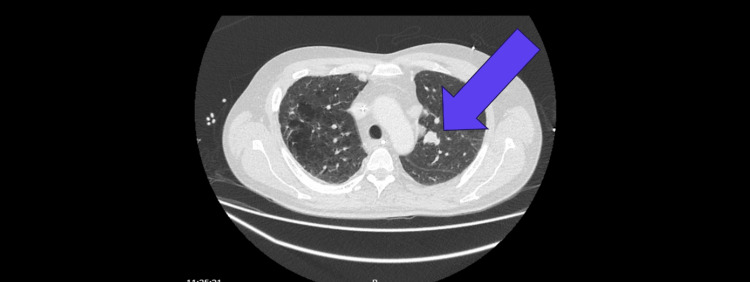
CT (chest, abdomen, and pelvis) showing mediastinal mass (4.2 cm × 1.4 cm × 2 cm) in the pre-vascular area with preserved fat planes.
